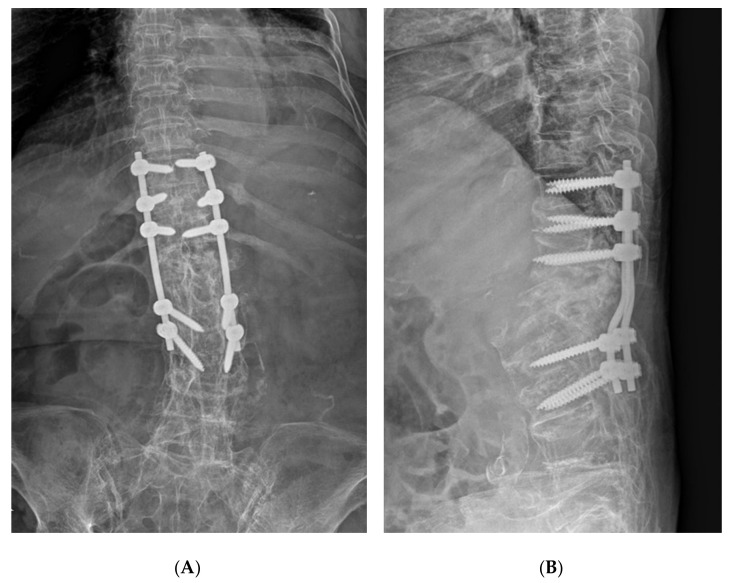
Postoperative plain radiograph (A,B).
